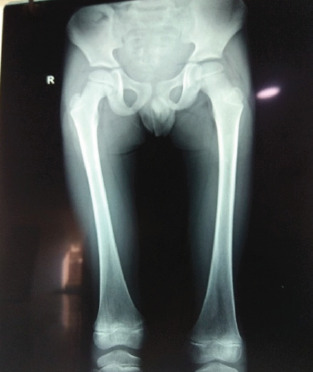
Plain radiograph showed no obvious signs of bony lesion.
